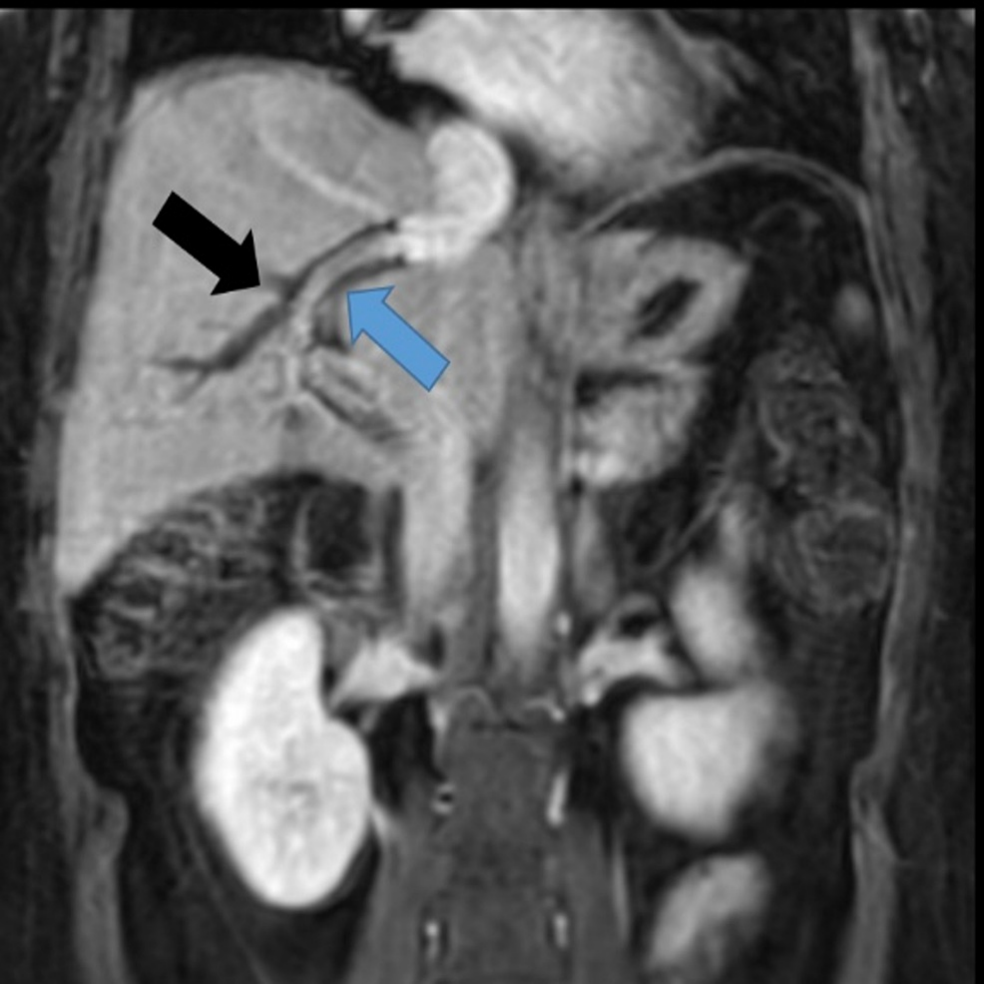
MRI abdomen, coronal post-contrast image, shows patent TIPS (blue arrow) and persistent complete thrombosis of all intrahepatic portal venous branches (black arrow). TIPS: transjugular intrahepatic portosystemic shunt
